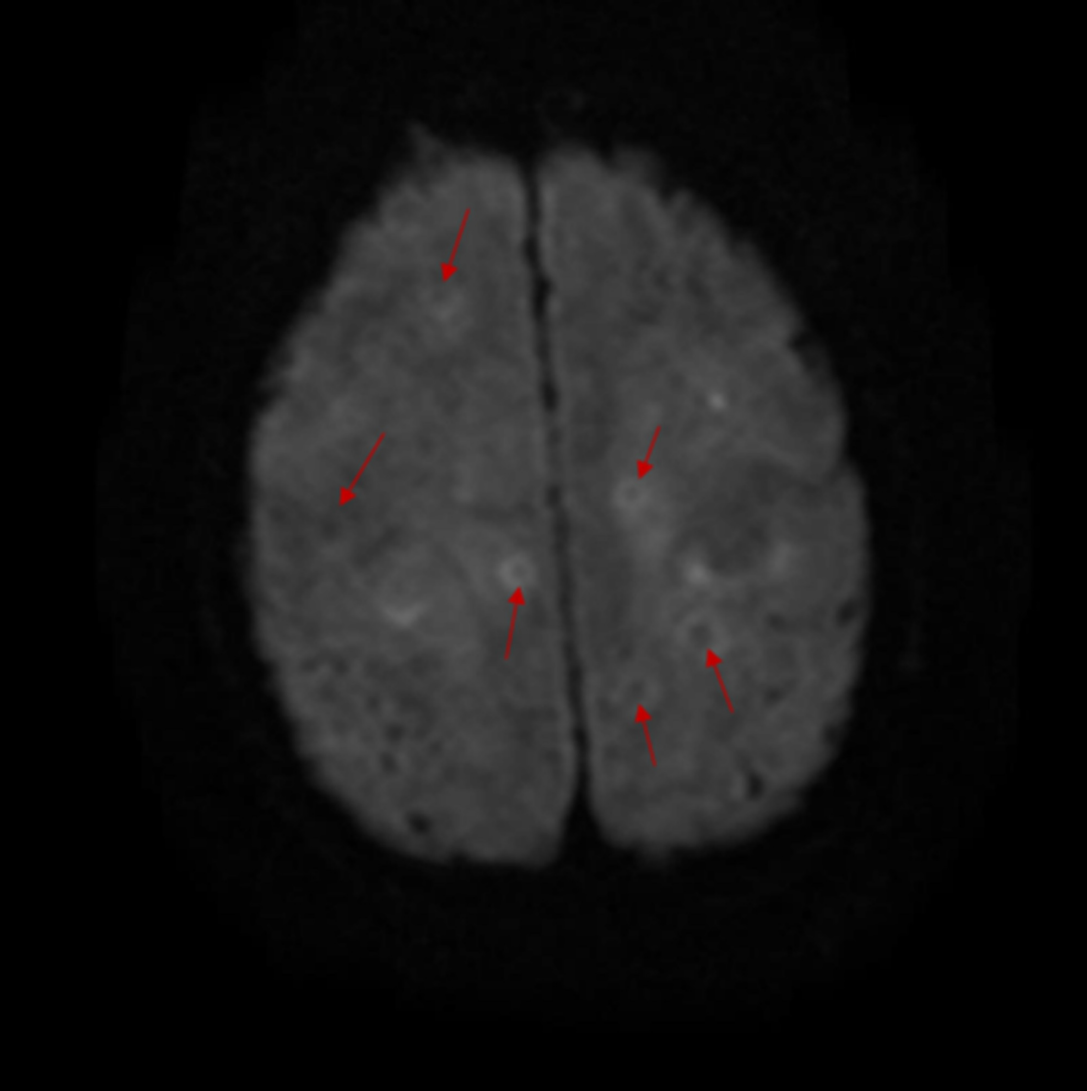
Axial DW MR imaging of the brain Multiple lesions are seen with central portion of these lesions showing low-intensity signal (red arrows). This finding is not consistent with restricted diffusion. DW, diffusion weighted. MR, magnetic resonance
